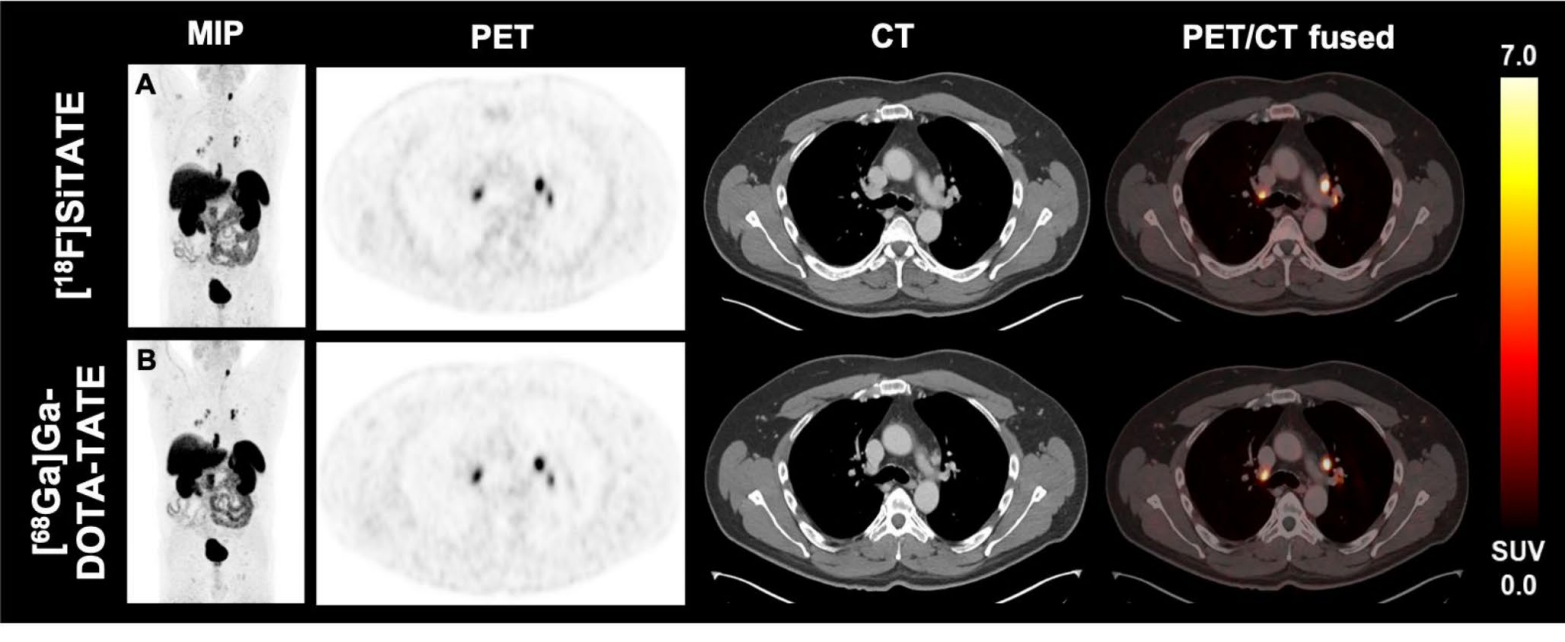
PET/CT imaging of a 52-year-old male patient diagnosed with MTC (patient 13) using the tracers [^18^F]SiTATE (A) and [^68^Ga]Ga-DOTA-TATE (B). The maximum intensity projection (MIP) and selected transversal levels of the baseline and follow-up PET are shown. The figure demonstrates hilar tumor manifestations. During the interval of 6 months the tumor volume increased by 14.6% and SUV_mean_ decreased by 5.7%, indicating stable disease
